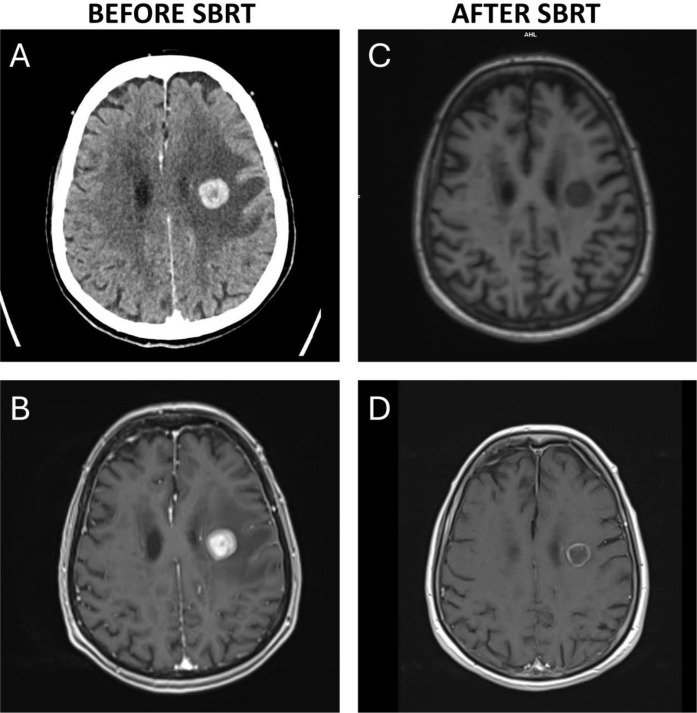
Contrast-enhanced brain CT examination [26.04.2024, (A)] and MRI CE-T1WI image [29.04.2024, (B)] demonstrating a 2 cm brain metastasis in the left frontal lobe, responsible for motoric aphasia. Follow-up MRI (09.08.2024), native T1WI (C) and CE-T1WI (D) revealed regression of peritumoral edema, in course of SBRT.
